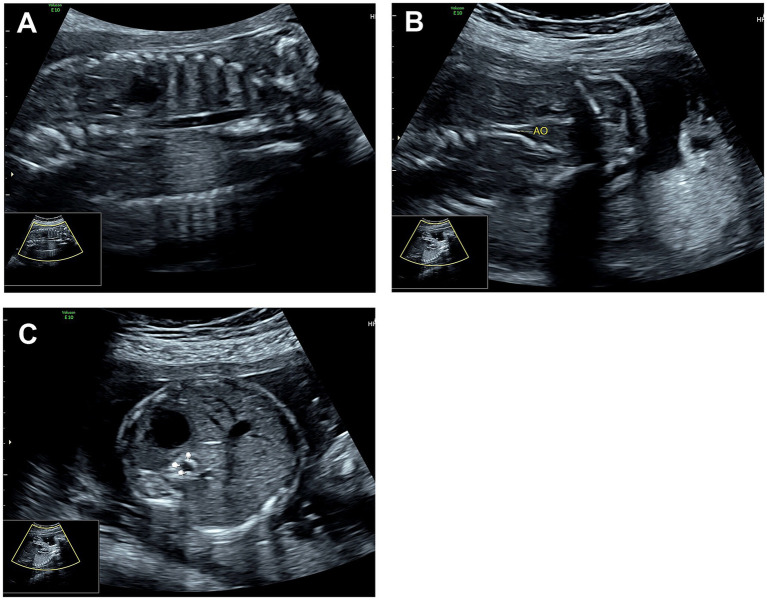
Fetal echocardiogram. (A) Calcification of the descending aortic wall, (B) Calcification of the descending aorta and iliac arteries, (C) The cross-sectional view of the descending aorta shows thickening and calcification of the vessel wall.
